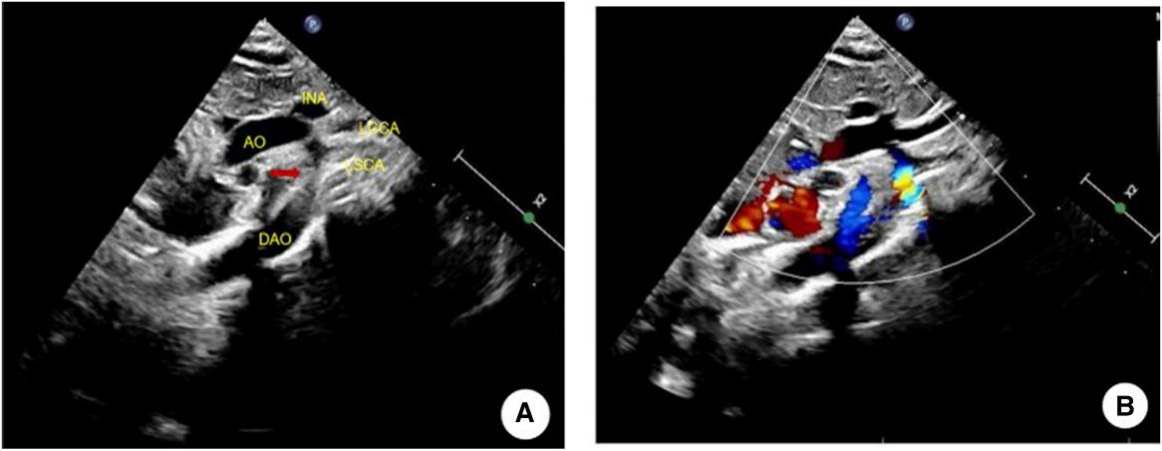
Echocardiogram. (*A*) Red arrow indicates the stenotic site in the descending aorta. (*B*) Fine blood flow through the stenotic site. AO, ascending aorta; INA, innominate artery; LCCA, left common carotid artery; LSCA, left subclavian artery; DAO, descending aorta.
